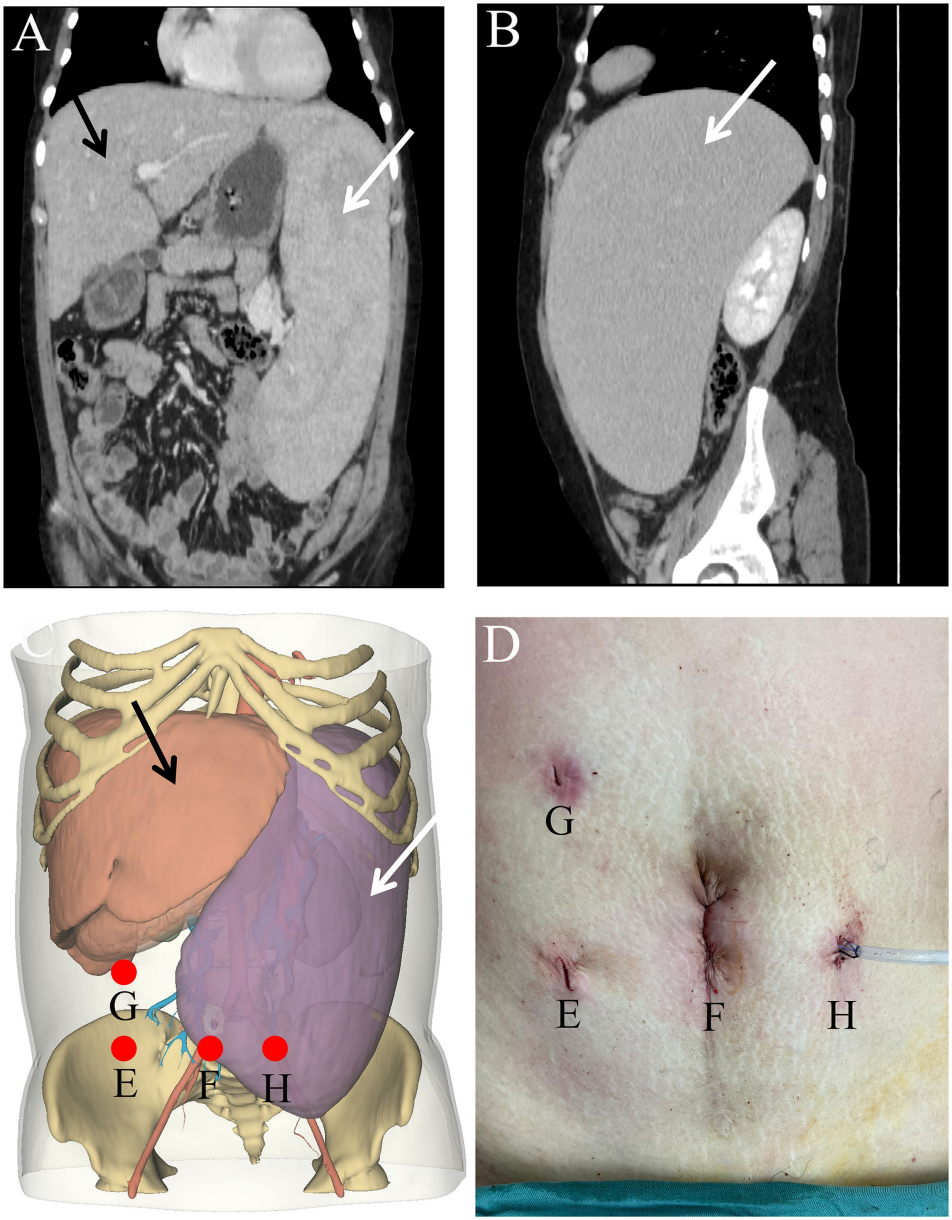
Coronal (A) and axial (B) views of the computed tomography images showing the spleen measures 27.3 cm in length, 6.0 cm in thickness, and 19.4 cm in width. Comparison of preoperatively planned trocar positions (C) and actual surgical sites (D). Location of surgical incision (E–H). The white arrow indicates the splenic artery, while the black markings denote the splenic vein.
